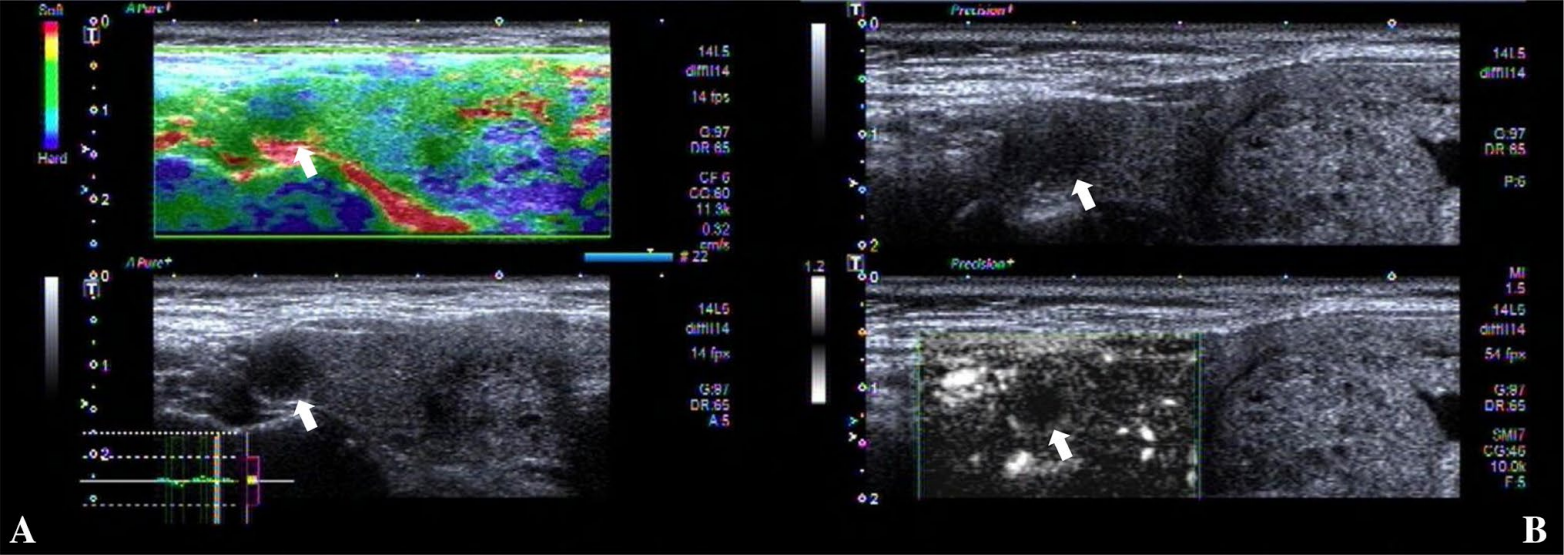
Images in a 51-year-old man who underwent routine. A 7 mm left thyroid nodule (arrows) with solid, hypoechogenicity, irregularity margin, wider than tall shape, no calcification was found at conventional ultrasound and assessed as malignant, ACR score of 6 and classified as TI-RADS category 4. A Type II was assigned at superb microvascular imaging. B A score of 2 was assigned at real-time elastography. This nodules was assessed as benign at multimodal ultrasound imaging. This thyroid nodule was diagnosed as nodular goiter at surgery
